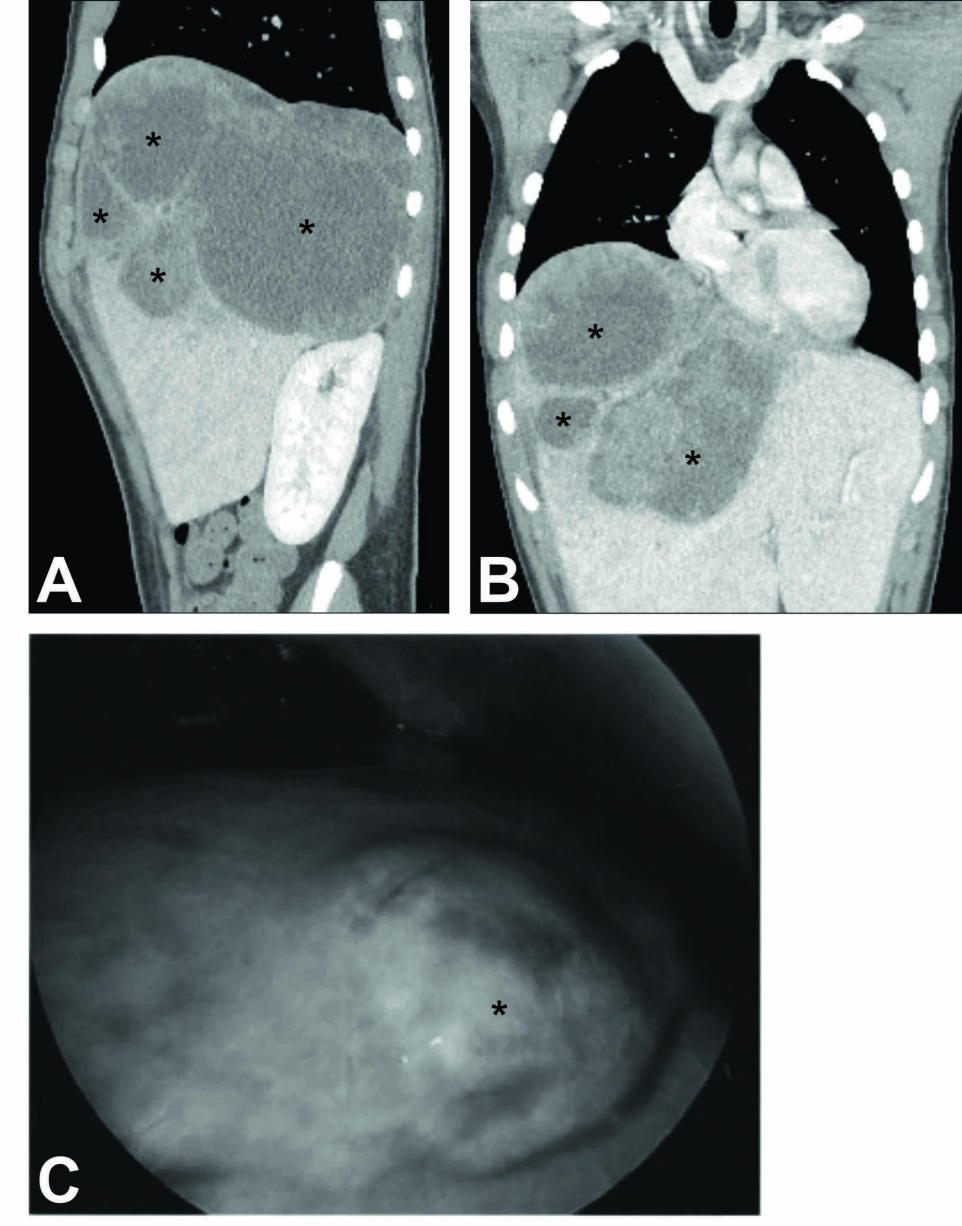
Visualization of the Hepatic Lesion (A, B) Computer tomography (CT) of the hepatic lesion. A CT scan of the abdomen and pelvis demonstrated a large (18 cm) cystic hepatic lesion shown in the sagittal (A) and coronal views (B), cysts are indicated with stars (*). (C) Intraoperative image during laparoscopic liver wedge biopsy. A portion of the hepatic mass that was biopsied can be seen (indicated by a star). The mass measured at least 18x16x14 cm and was centered within the right hepatic lobe.
